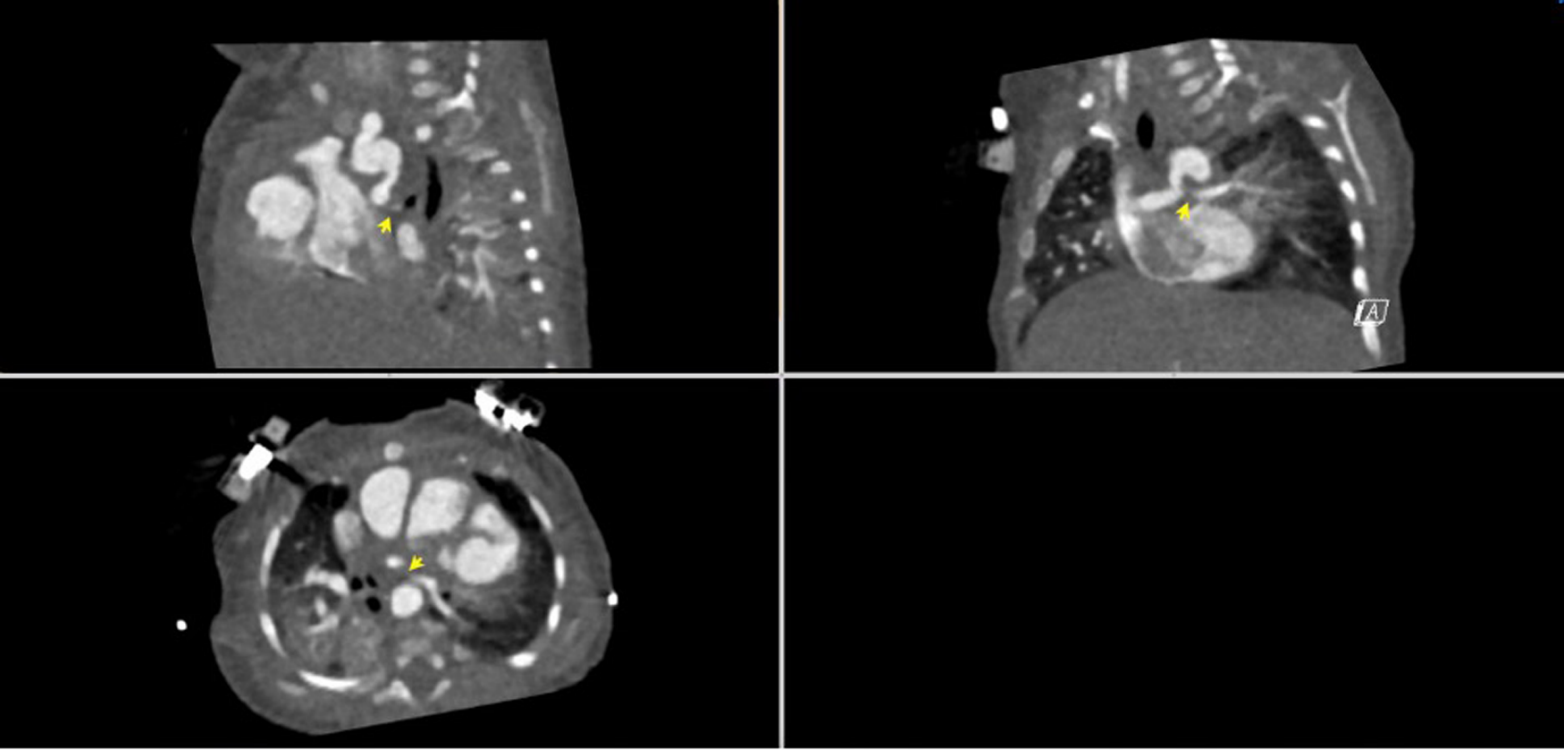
CT angiogram demonstrating the aorta-to-pulmonary central shunt that originates from the under-side of the distal transverse artic arch immediately underneath the origin of the left subclavian artery. The proximal LPA is not visualized. The LPA reconstitutes distally and is diffusely hypoplastic.
